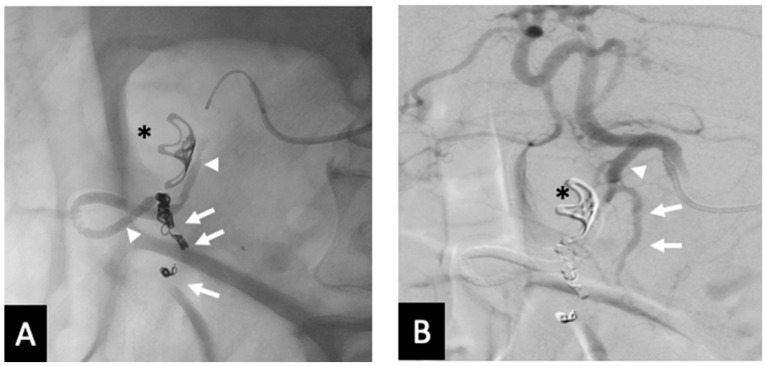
Rebleed example of unembolized SDA—(A) Post-embolization radiograph showing 3 mm coils in the proximal RGEA and inferior pancreaticoduodenal artery (arrows). Continuous column of Ta-OCE extending from the coil pack in the proximal RGEA to the proximal GDA (arrow head). An endoluminal clip placed during endoscopy is also demonstrated (*). (B) DSA with contrast injection from the CHA shows patent SDA (arrow) and proximal GDA (arrowhead). This patient required a second embolization to address the SDA. Image obtained from patient’s chart. Source: NK.
